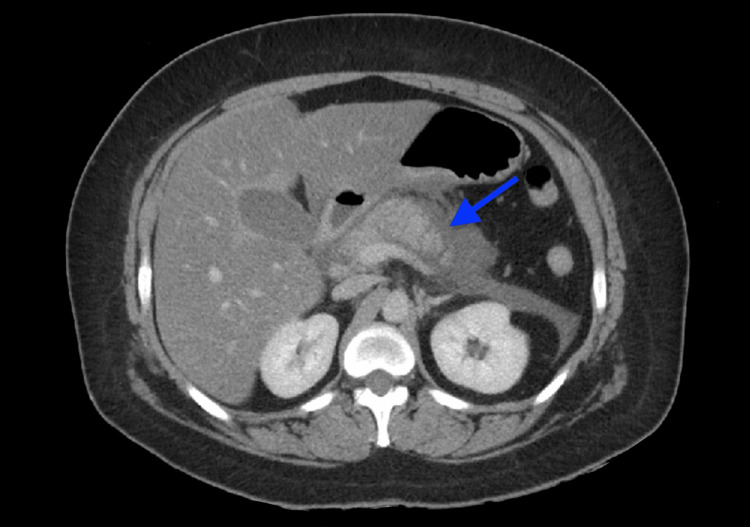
Computed tomography (CT) scan of the abdomen revealing areas of necrosis (blue arrow) indicative of acute necrotizing pancreatitis.
